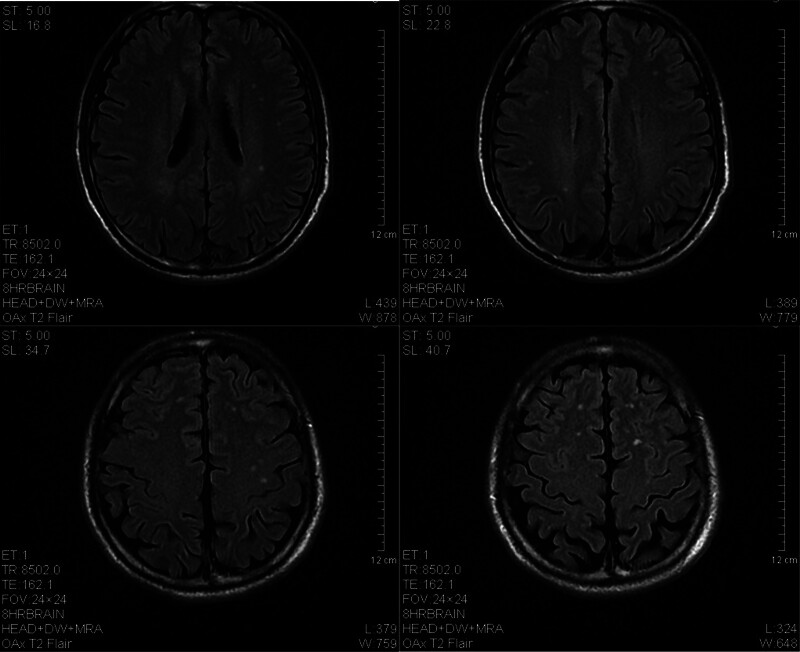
Brain magnetic resonance imaging of the patient (T2 Flair).
